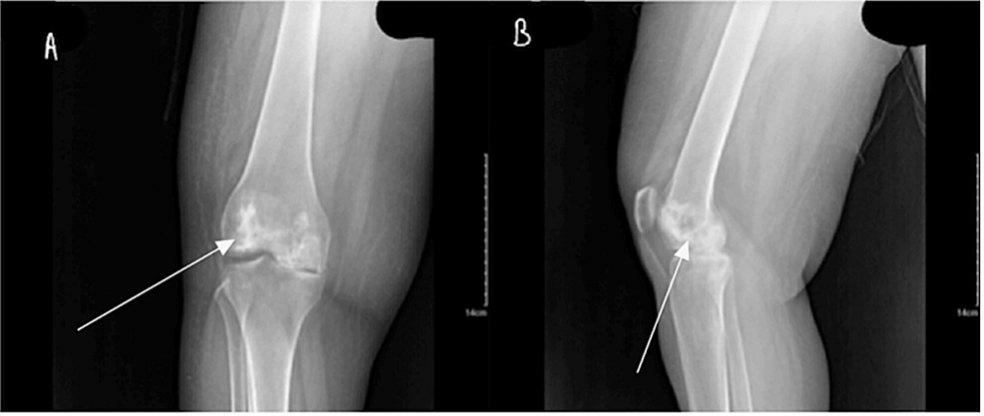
Anteroposterior (A) and lateral (B) radiographs of the right knee. Arrows indicate the area of avascular necrosis.
